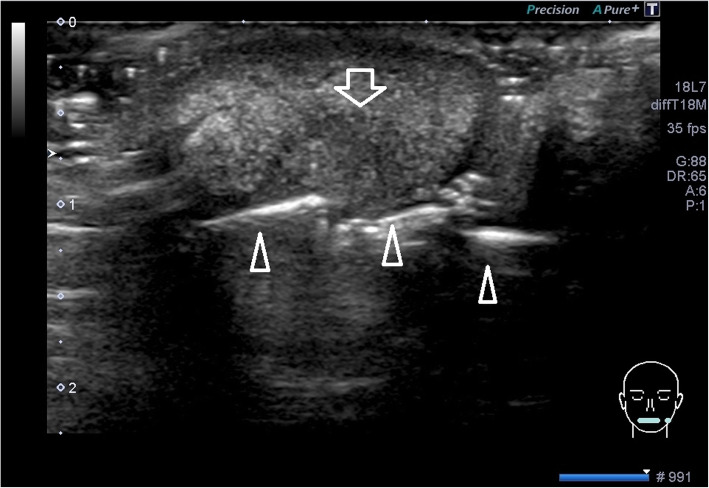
Sonogram of the lesion on the lower lip. A gray-scale and transverse sonogram of the lesion obtained with the patient’s mouth closed. The shape of the lesion was almost oval. A heterogeneous tumor-like lesion of 17 × 11 × 12 mm in size was observed in her left lower lip. The ulcerative lesion was hypoechoic (*white arrow*). The mandibular teeth are indicated with *white arrowheads*
